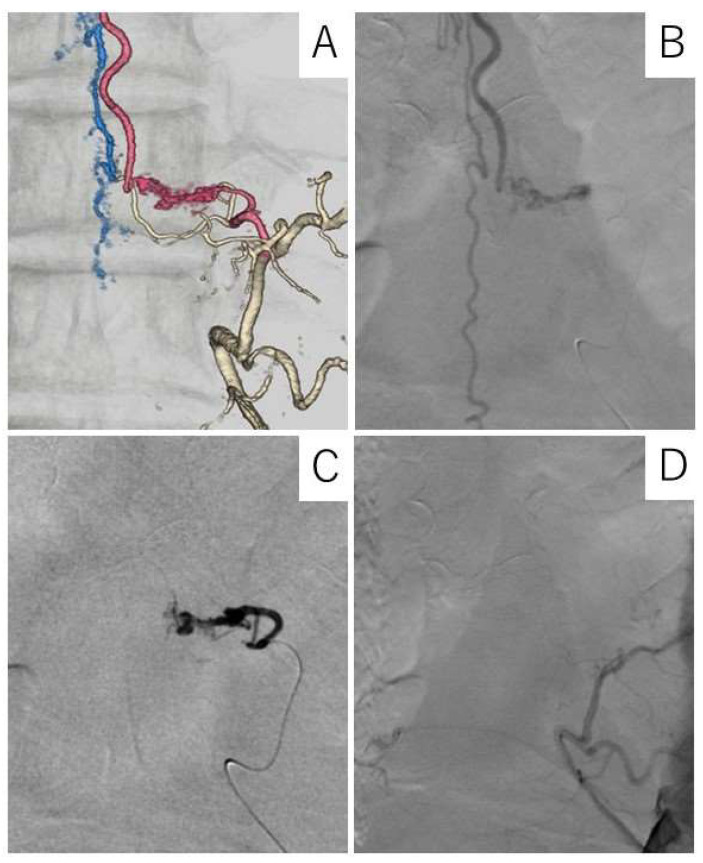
Three-dimensional digital subtraction angiographic (DSA) images and spinal X-ray of a patient with dural arteriovenous fistula (DAVF). (A) Anteroposterior DSA view of the right Th-9 and -10 segmental arteries; DAVF, intradural arterialized vein, and dilated perimedullary veins can be seen; (B) anteroposterior DSA view of right Th-9 segmental artery displaying a shunt lesion from selective catheterization of right Th-9 radicular branch and a tangle of arterialized veins in the central spinal canal; fistula can be seen at right Th-9 nerve sleeve; (C) post-treatment X-ray showing the presence of N-butyl cyanoacrylate (NBCA); (D) post-treatment anteroposterior DSA view of right Th-9 and -10 segmental arteries showing disappearance of the spinal DAVF.
